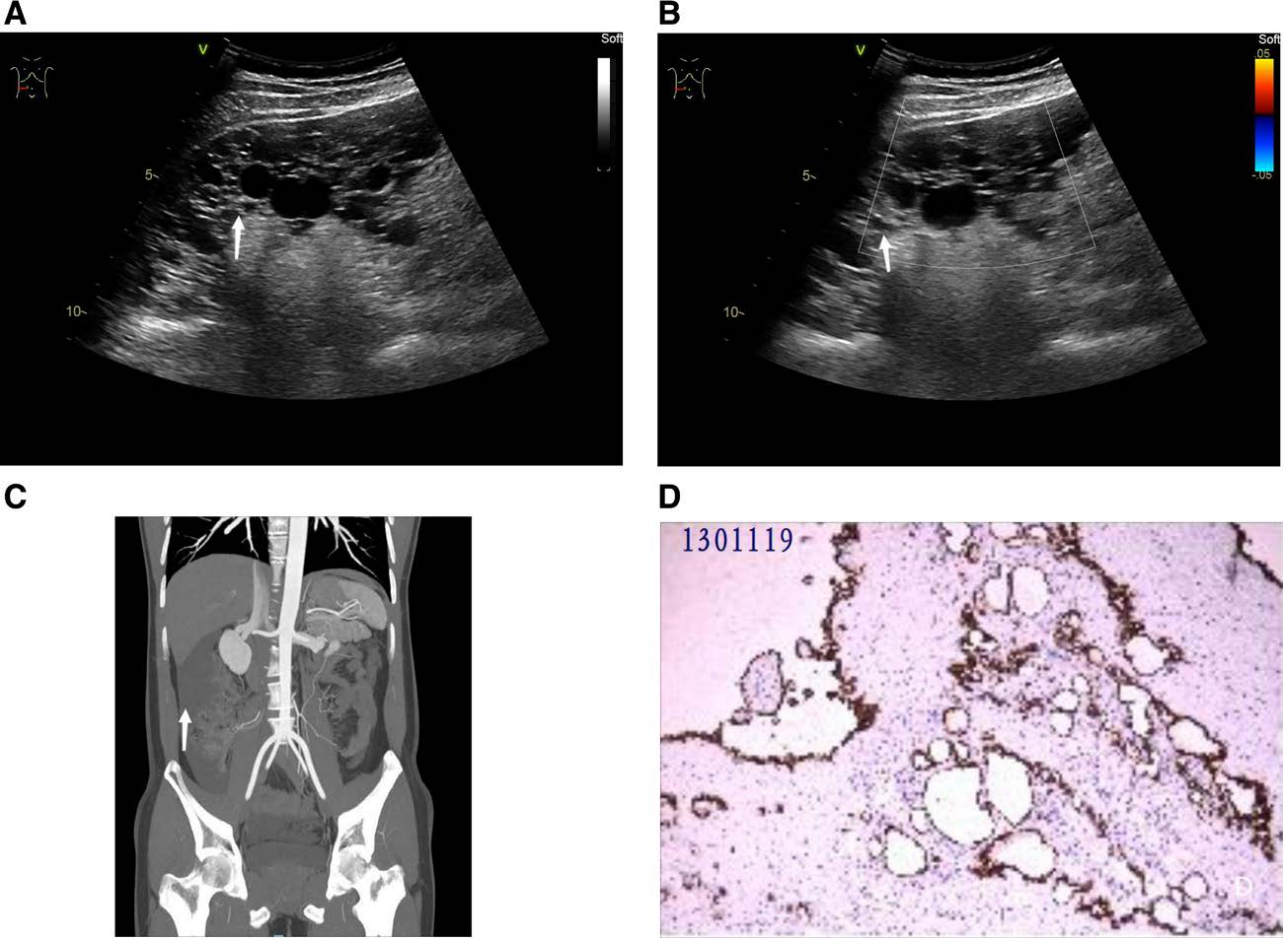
A 34-year-old male patient with Multicystic mesothelioma of the omentum. (A) Ultrasound of an abdominal mass (arrow); (B) No obvious blood flow signal (arrow) Color Doppler ultrasound image in abdominal mass; (C) CT of an abdominal mass (arrow); (D) Pathological map of an abdominal mass (HE, ×200). CT = contrast-enhanced computed tomography.
